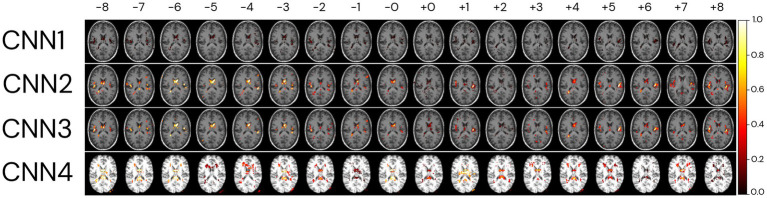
Relevant regions for the brain age prediction based on 1% of the salience maps of individuals with an average age of 65 years overlayed to a random T1w MRI sample for each of the four CNN models. The individuals were randomly selected in the test dataset used in CNN1, according to their brain age difference and proximity to the average age of our sample (65 years). Each row represents the salience maps of one CNN model, where the columns show the same individual’s salience map in each of the four models. The SmoothGrad method was used to generate all salience maps. The brain age difference is shown in the top row of the image, going from −8 to +8 years. Overlaid salience map colours are normalized for each individual between 0 and 1.
